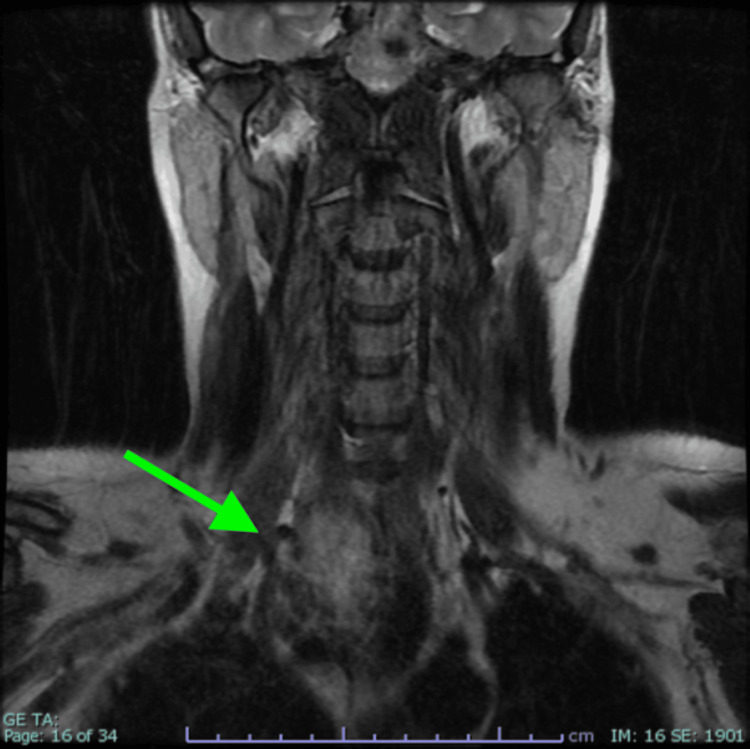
Initial MRI demonstrating a right sided thyroid mass (arrow) with compression and midline shift of the trachea.
